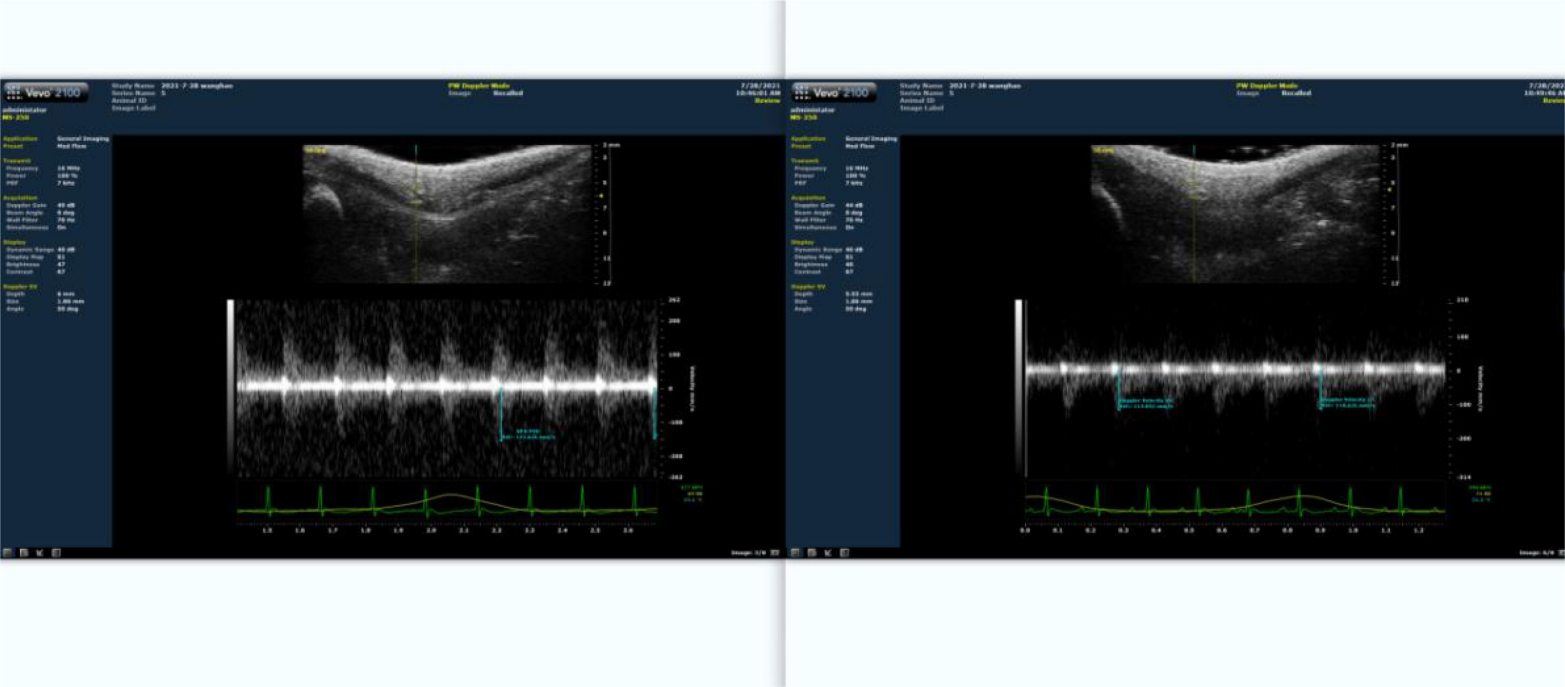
Proportion of blood flow restriction detected by ultrasound. The left panel depicts the situation prior to blood flow restriction. The right panel represents the results of blood flow limitation.
